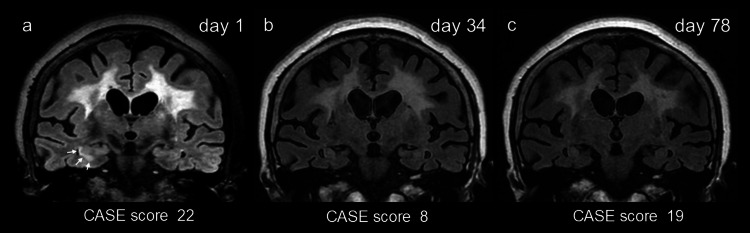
Chronological changes on brain MRI and Clinical Assessment Scale for Autoimmune Encephalitis (CASE) score. Note increased signal on fluid-attenuated inversion recovery (FLAIR) in the right hippocampus on the day of admission (a, arrows), but no apparent high signal on the follow-up MRIs obtained after recovery of symptoms (b) and even on the day of readmission due to clinical relapse (c). Panels a, b, and c were obtained on the day of admission (day one), after two cycles of intravenous high-dose methylprednisolone and four rounds of plasma exchange (day 34), and on the day of readmission due to clinical relapse (day 78), respectively. Panel (a) was acquired with a 3 Tesla scanner, while panels (b) and (c) were performed on a 1.5 Tesla scanner. The diffuse FLAIR high intensity in the white matter was considered as pre-existing ischemic changes because this finding was already seen on the MRI scans taken 10 months before the presentation to our facility (data not shown).
